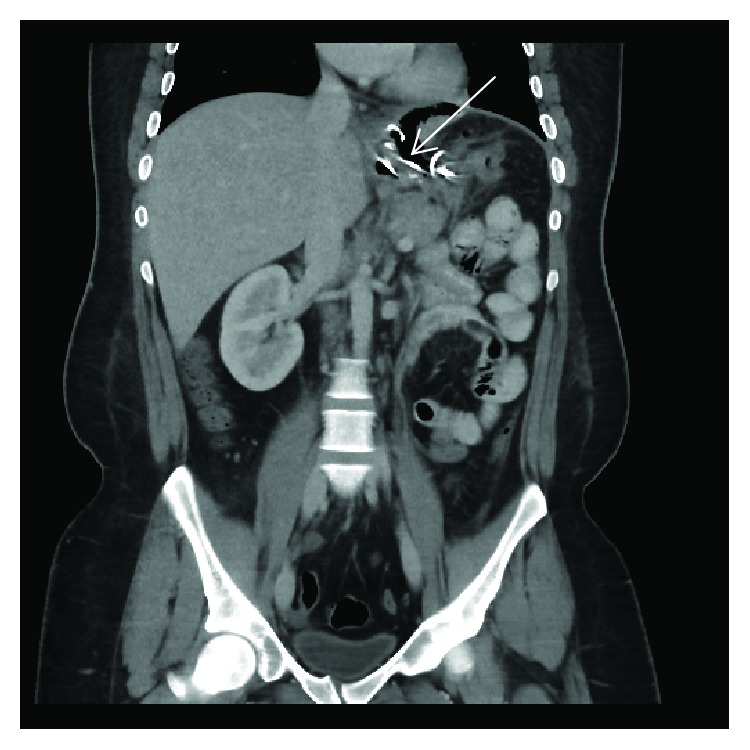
Abdominal computed tomographic scan with intravenous contrast shows no leakage, and as compared to the previous study, the collection regressed after putting a stent. Arrow: displaced stent into the collection.
